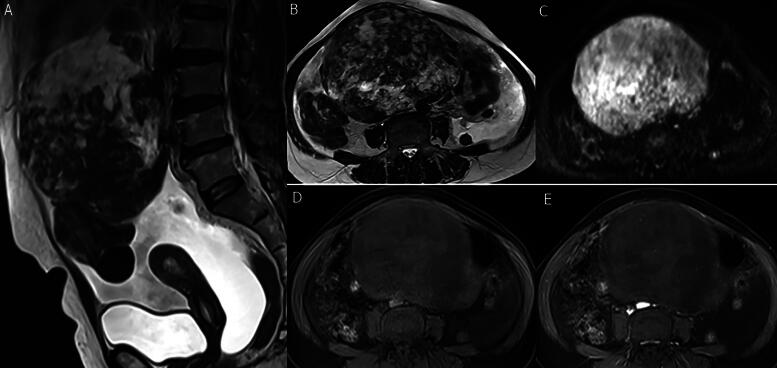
Huge right ovarian Fibrothecoma in a 67-year-old woman presented with abdominal enlargement. (A and B) sagittal and axial T2-WI revealed a large pelvi-abdominal lobulated mixed right ovarian lesion of mixed signal with predominant hyperintensity of cystic changes, associated endometrial thickening/hyperplasia, and marked ascites. (C) Axial DWI (high *b* value = 1000) revealed a predominant hyperintense signal (edema). (D and E) Axial fat-suppressed pre- and postcontrast T1 images revealed mild enhancement of the mass.
